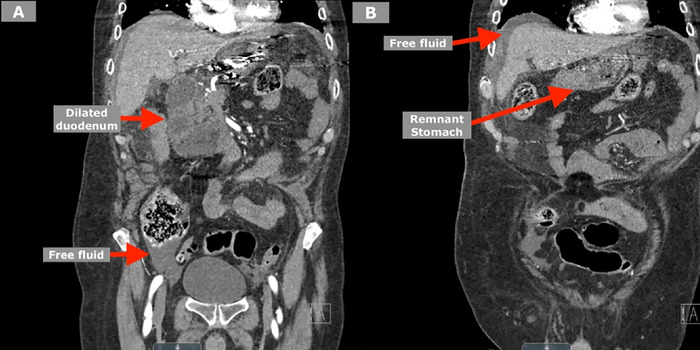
Computed tomography scans in the coronal plane show (A) the dilated duodenum with free fluid beneath the colon and (B) the dilated remnant stomach with free fluid surrounding the liver.
